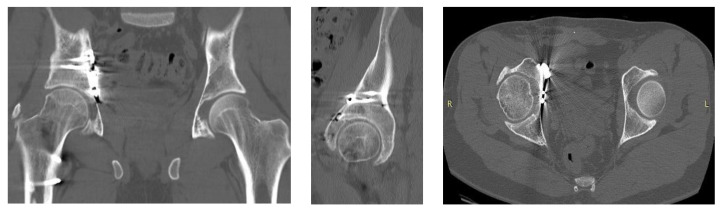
Postoperative CT scan of a clinical case exhibited in coronal (left), sagittal (middle), and axial (right) views and demonstrating excellent anatomical reduction.
